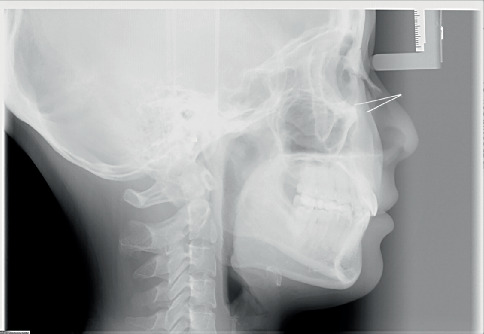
Posttreatment cephalometric x-ray image.
